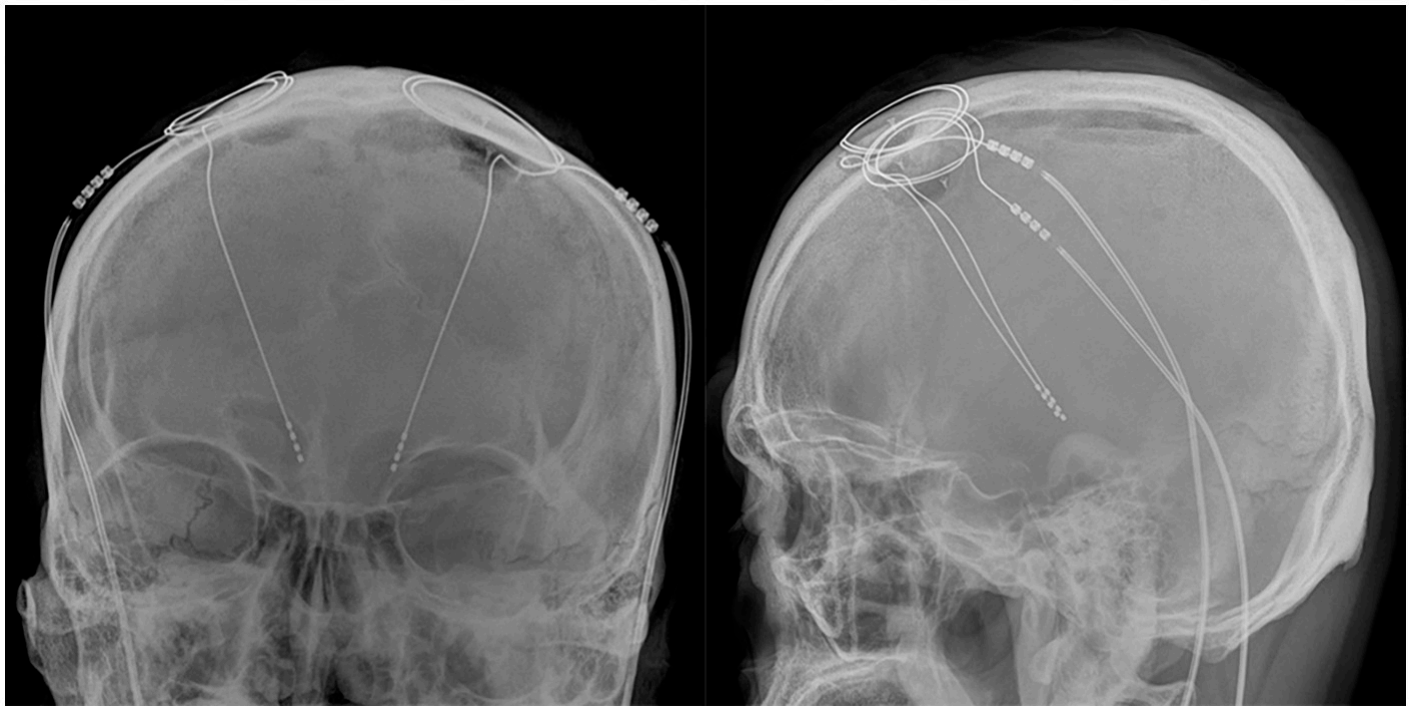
A representative patient’s postoperative intracranial leads on plain radiographs: The leads are obviously bent.
